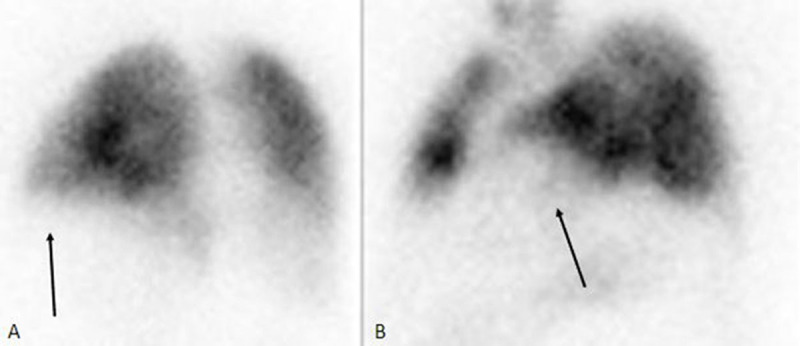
A lung scintigraphy including only perfusion (Q) sequences, after injection of 4.5 cCi of MAA-Tc99m was realized. Six incidences were performed. In two incidences there was a perfusion defect in the territory of the sub-segmental inferior lingula artery that corresponded exactly to the pulmonary infarction seen on the thoracic CT scan. A diagnosis of pulmonary embolism was made based on PISAPED criteria.
